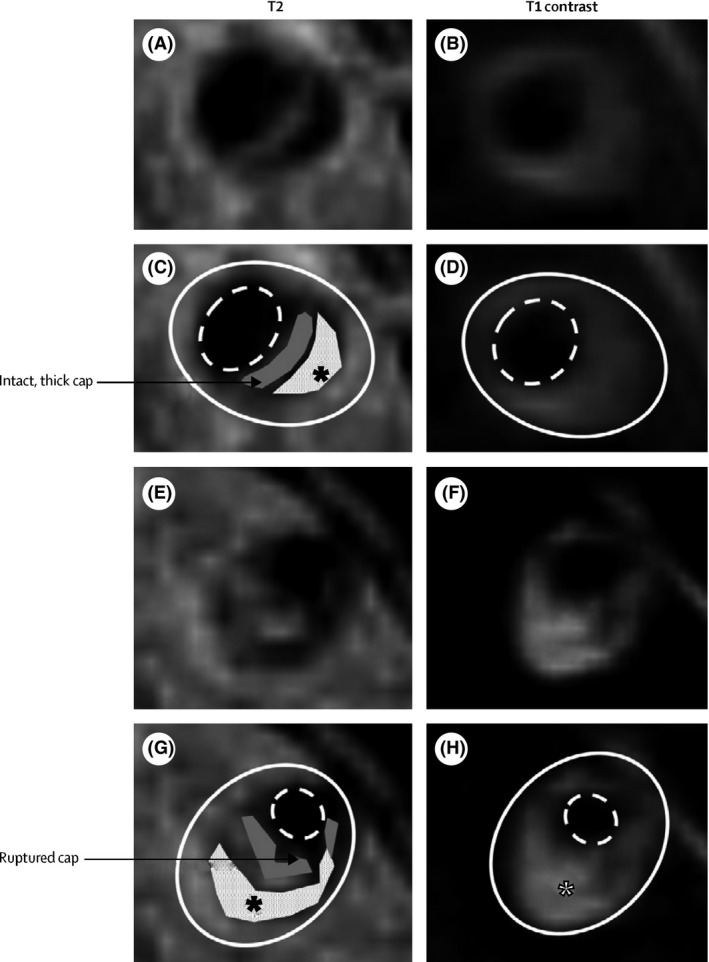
High‐resolution MRI of vertebral artery stenoses with plaque components Panels A–D show T2‐weighted and T1 postcontrast images (panels C and D have plaque components marked) of a crosssection of a vertebral artery plaque with a thick, intact, fibrous cap (gray) and lipid core (white with black asterisk). Panels E–H show T2‐weighted and T1 postcontrast images (panels G and H have plaque components marked) of a cross section of a vertebral artery plaque with a ruptured fibrous cap (gray) and lipid core (white with black asterisk), which enhances with contrast (white asterisk) and is also indicative of plaque rupture. The solid white line shows the outside vessel wall and the dashed white line the lumen “Reprinted from Holmstedt et al. (2013) with permission from Elsevier”
